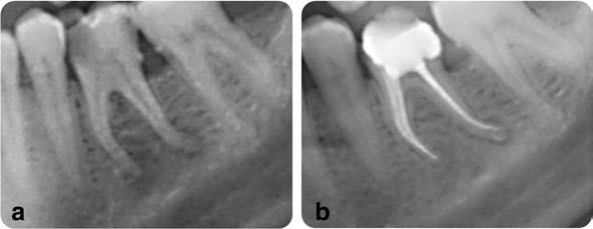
A representative x-ray image of incomplete healing, a before RCT, b a-year follow-upNo healing (F): apical lesion was enlarged/unchanged, or a new apical lesion observed on recall radiograph (Fig. 3).
